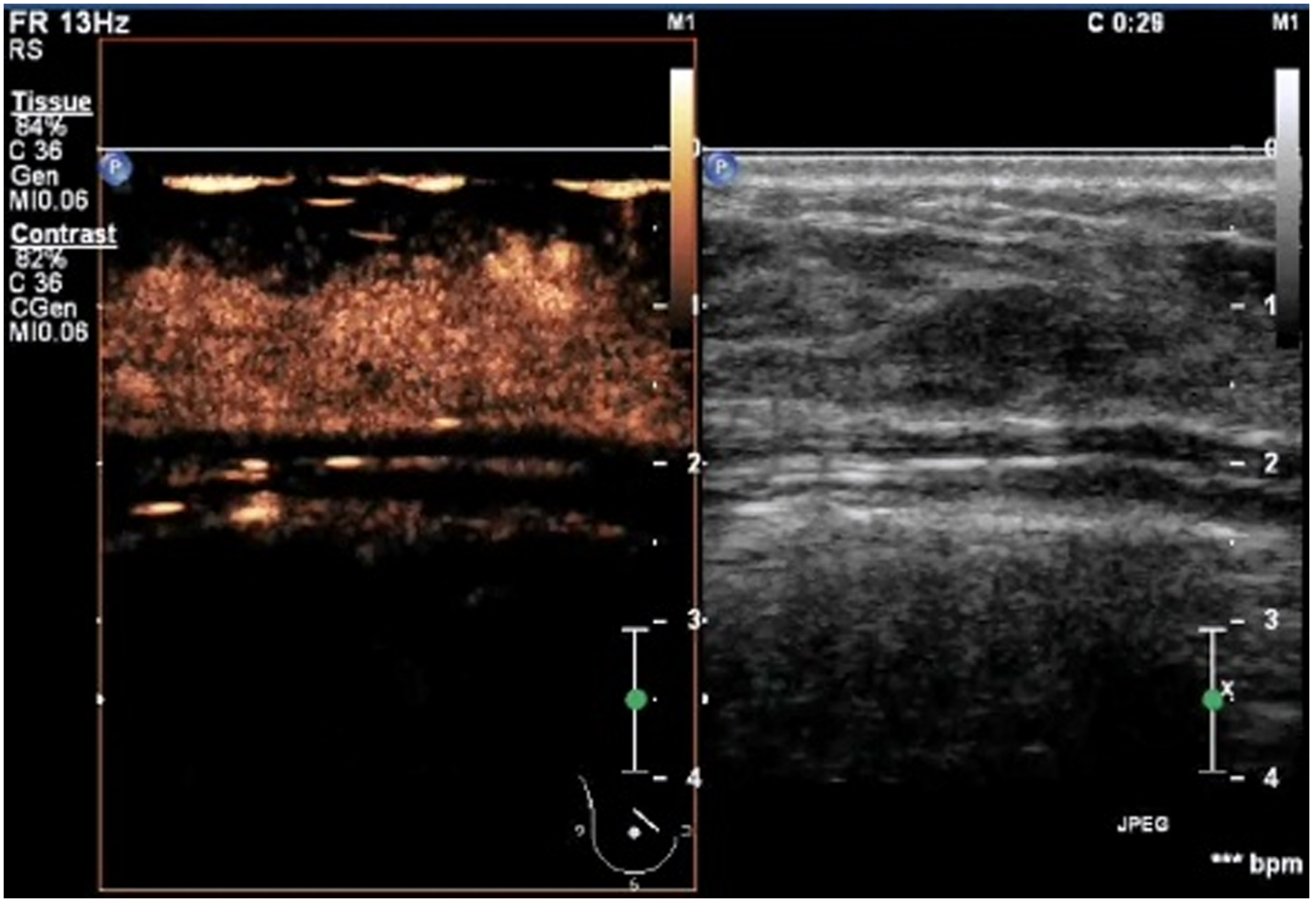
A lesion with a score of 2 by breast contrast-enhanced ultrasound. The lesion displayed iso- and synchronous enhancement with the surrounding tissue, without a clear outline in the contrast-enhanced image.
